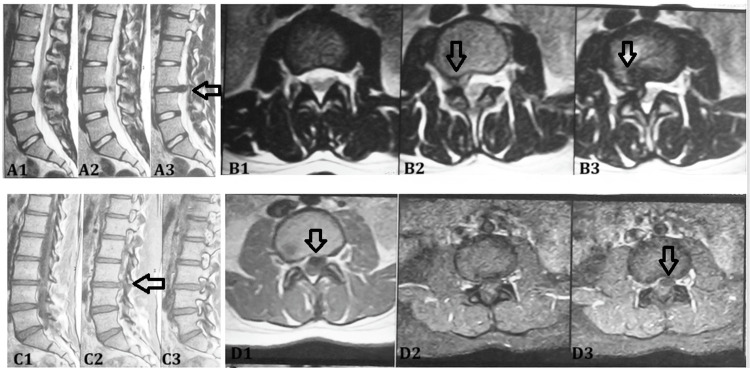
Sagittal (A1-A3) and axial (B1-B3) T2-weighted MRI at the L4-L5 level (lumbarised S1) demonstrate an extruded intervertebral disc herniation with posterior migration, causing the significant compression of the thecal sac and displacement of adjacent nerve roots; the areas of interest are indicated by arrows in A3, B2, and B3. Contrast-enhanced sagittal (C1-C3) and axial (D1-D3) T1-weighted MRI at the same level show peripheral ring enhancement surrounding the extruded disc fragment, suggestive of inflammatory neovascularization and granulation tissue formation; arrows highlight the areas of interest in C2, D1, and D3, consistent with an ongoing resorptive process. A3, B2, B3 (T2-weighted images): arrows indicate the extruded disc fragment that has migrated posteriorly, causing the significant compression of the thecal sac and displacement of the adjacent nerve roots. C2, D1, D3 (post-contrast T1-weighted images): arrows highlight the peripheral ring enhancement surrounding the extruded disc fragment, representing vascularized granulation tissue and inflammatory changes consistent with disc resorption. MRI: magnetic resonance imaging
